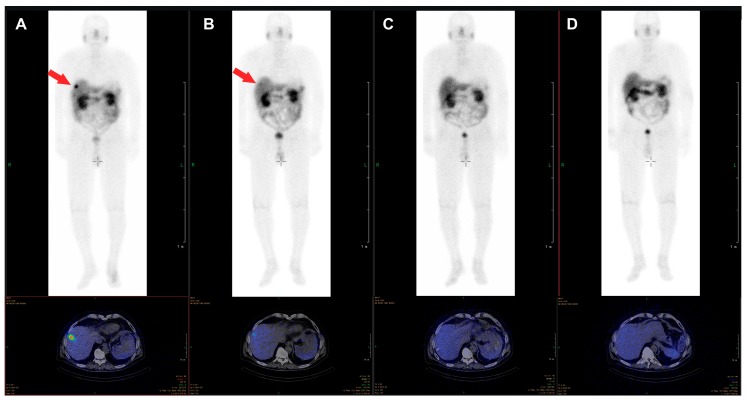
Example of a 76-year-old male patient with pancreatic NET, first diagnosed in 2012, with an initial ki-67 index of 15% who underwent four cycles of treatment with ^177^Lu-DOTATATE (cumulative dose 28.8 GBq) between October 2017 and July 2018, due to progression of bilobar liver metastases. The liver metastasis clearly visible in the post-therapy scan of the first cycle (A) is just barely visible in the scan of the second cycle (B) and not visible anymore in the scans of the third (C) and fourth (D) cycle. In the PET/CT images acquired six and twelve months after the last cycle, no tumor tissue was identified.
